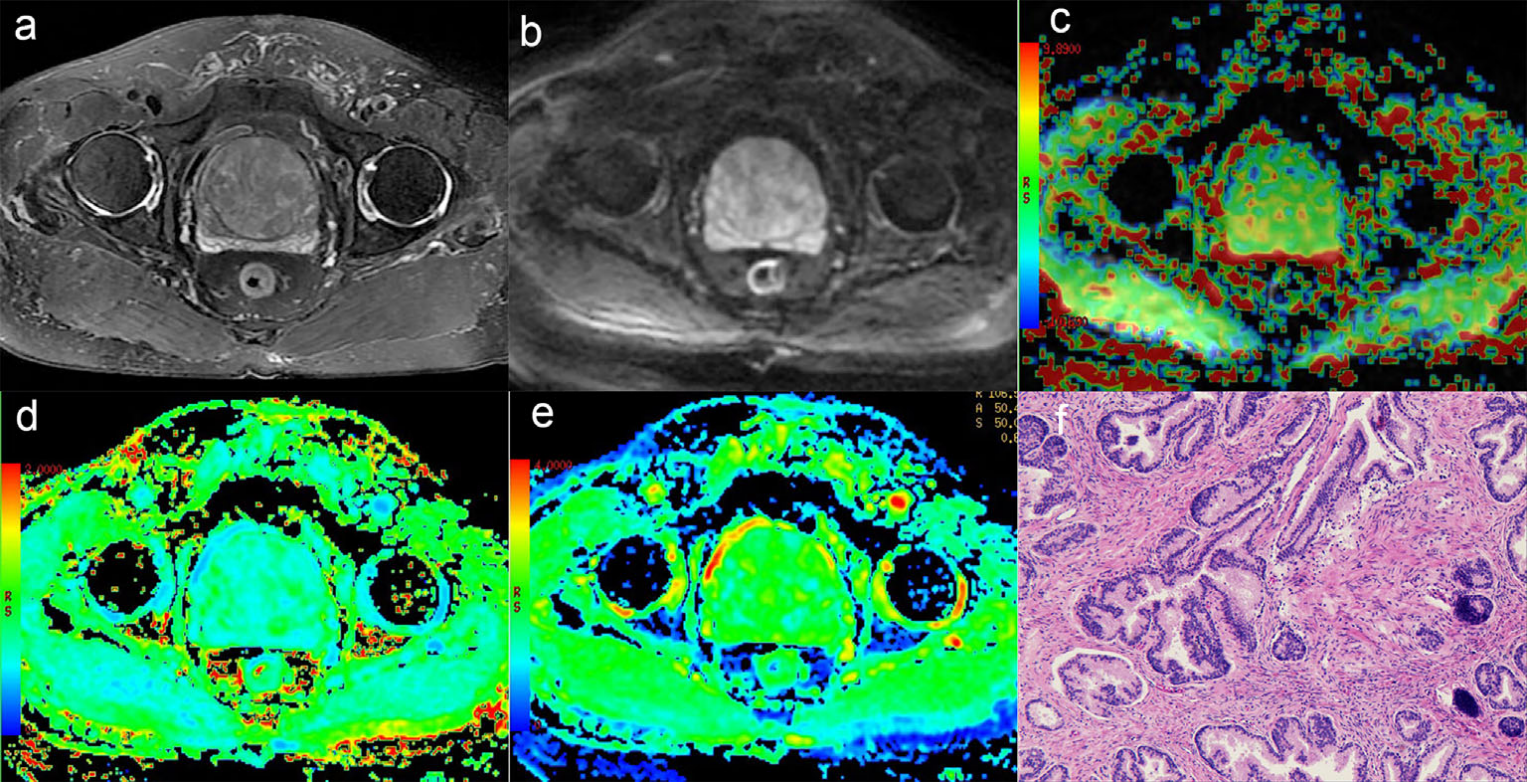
A 75-year-old man with BPH. (A) T2-weighted image, (B) Diffusion-weighted image, (C) APT pseudo-colored map, (D) Mean kurtosis pseudo-colored map, (E) Mean diffusivity pseudo-colored map, (F) pathological image.
